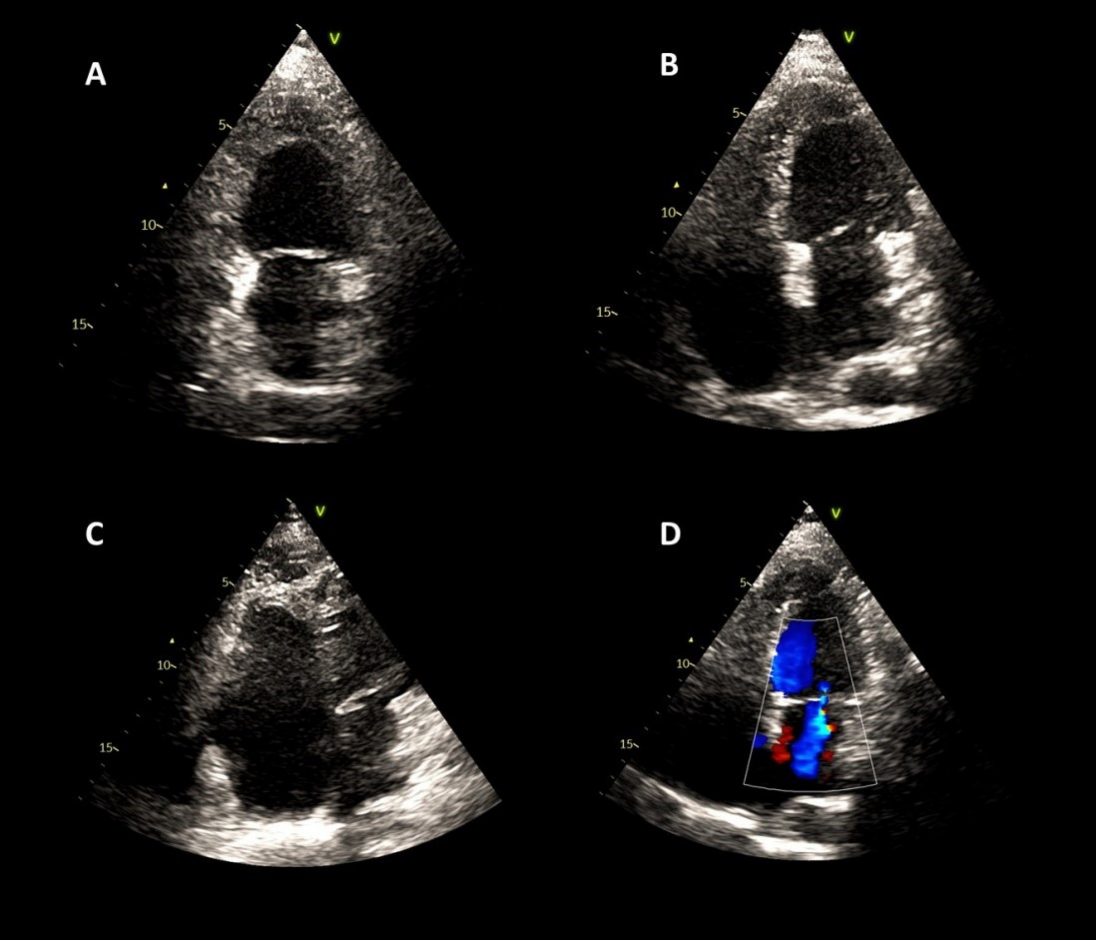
Imagens de ecocardiograma transtorácico de um paciente diagnosticado com SHE e obliteração apical do ventrículo esquerdo no corte apical de 2 câmaras (A), corte apical de 4 câmaras (B) e do ventrículo direito no corte apical modificado para avaliação do VD (C). Observa-se insuficiência mitral de grau discreto ao Doppler colorido.
